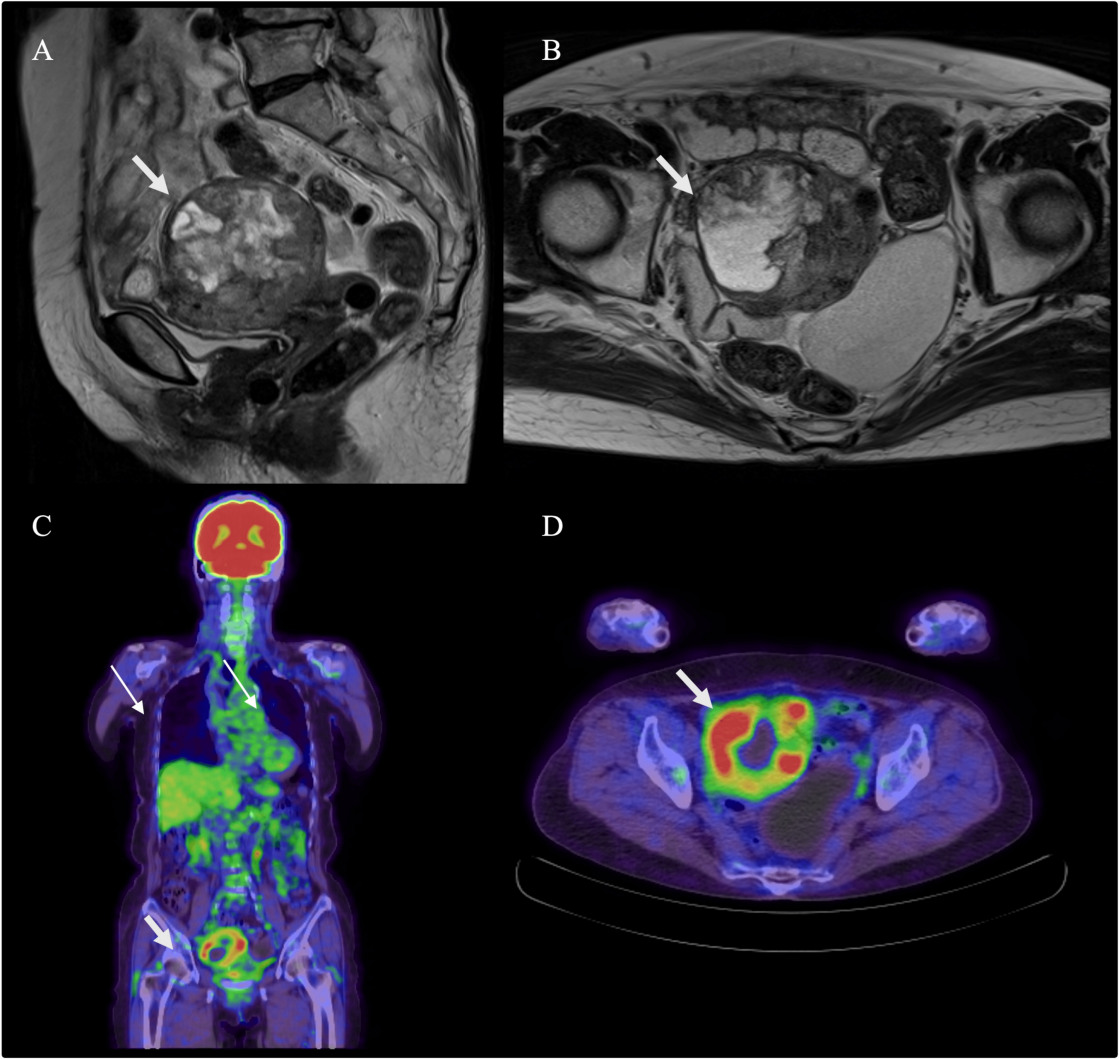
Preoperative MRI and FDG-PET/CT imaging of the uterine tumor A)  Sagittal T2-weighted MRI shows a 7.7 cm intramural uterine mass with heterogeneous signal intensity within the myometrium (thick white arrow).
